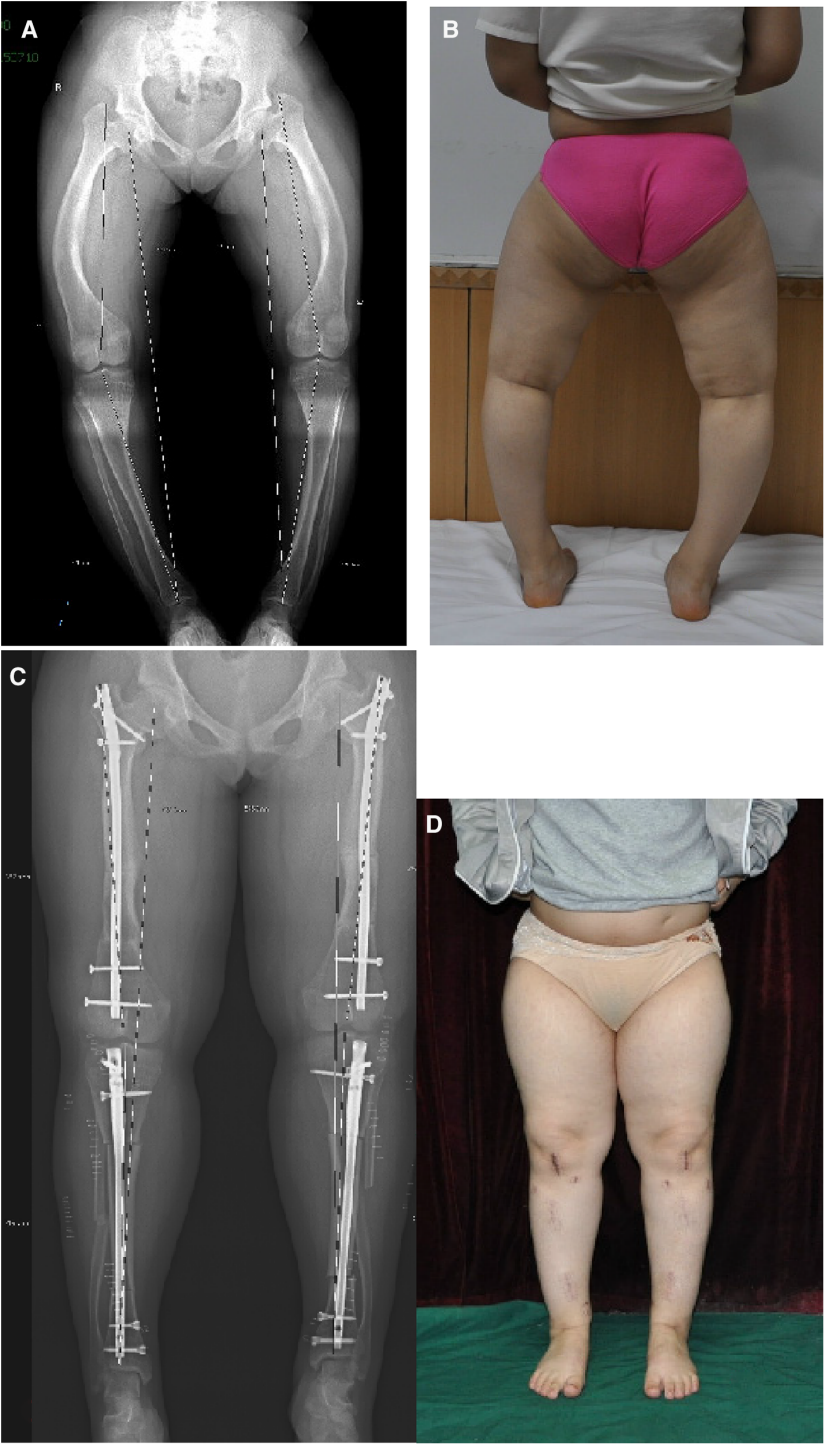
Typical case. A and B are the preoperative full-length radiograph and body image of the patient; C and D are the postoperative radiograph and body image of the patient.
